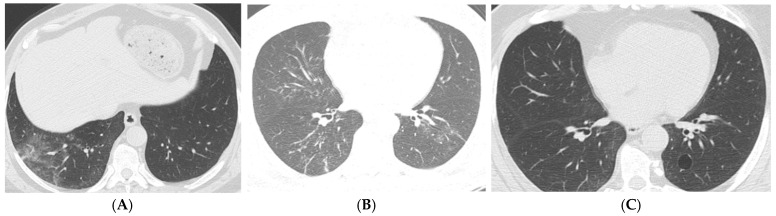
Axial CT scans of a 68-year-old male patient with mild to moderate COVID-19. The CT obtained 4 days after the onset of symptoms shows a CT severity score of 8; however, the patient was admitted to the intensive care unit and placed on mechanical ventilation 7 days later. (A) The scans reveal peripheral ground-glass opacities, consolidation areas, traction bronchiectasis, and bronchiolectasis in the posterior basal segment of the right lower lobe. (B) At the mid-zone level, linear patchy consolidation areas, mild ground-glass densities, and slight bronchiectasis are observed. (C) The follow-up CT performed 13 months later shows a cyst in the anterior basal segment of the left lower lobe, which was not evident in the previous examination.
